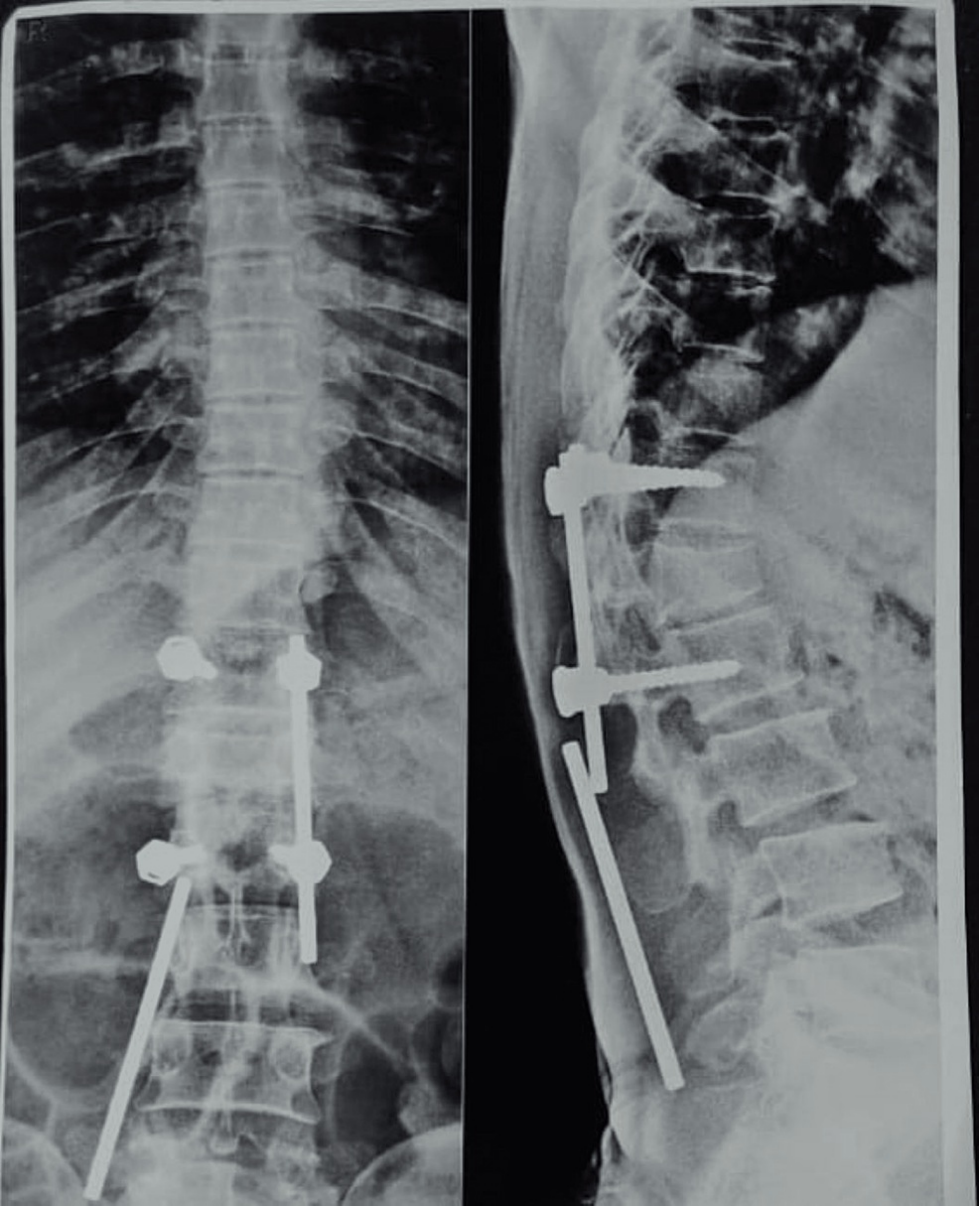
X-ray of vertebral column of the patient The X-ray image shows the fixation of implants in the vertebral column from posterior and lateral views.
